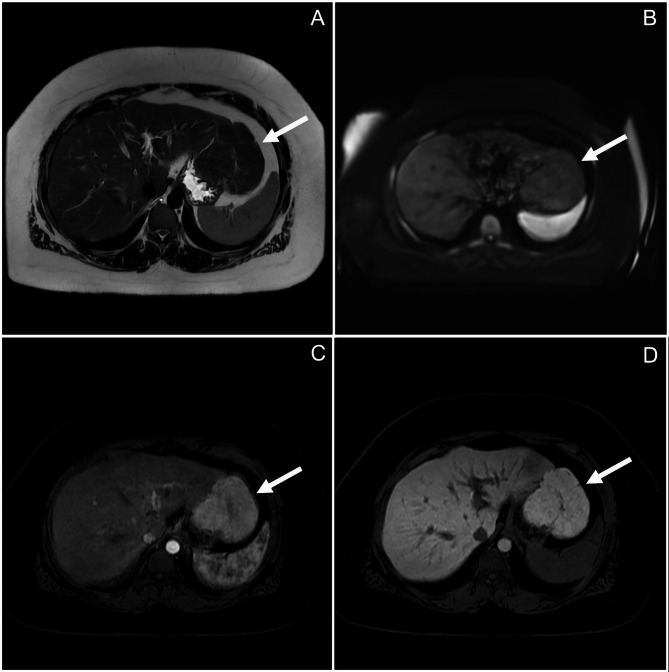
MRI imaging indicated pedunculated focal nodular hyperplasia. The lesion is isointense compared to surrounding hepatic tissue on T2-weighted MRI (A) and Diffusion-weighted imaging (DWI, B). With injection of Eovist contrast, the lesion became enhanced during the arterial phase (C), and remained enhanced after 20 min (D), findings that are characteristic for FNH.
